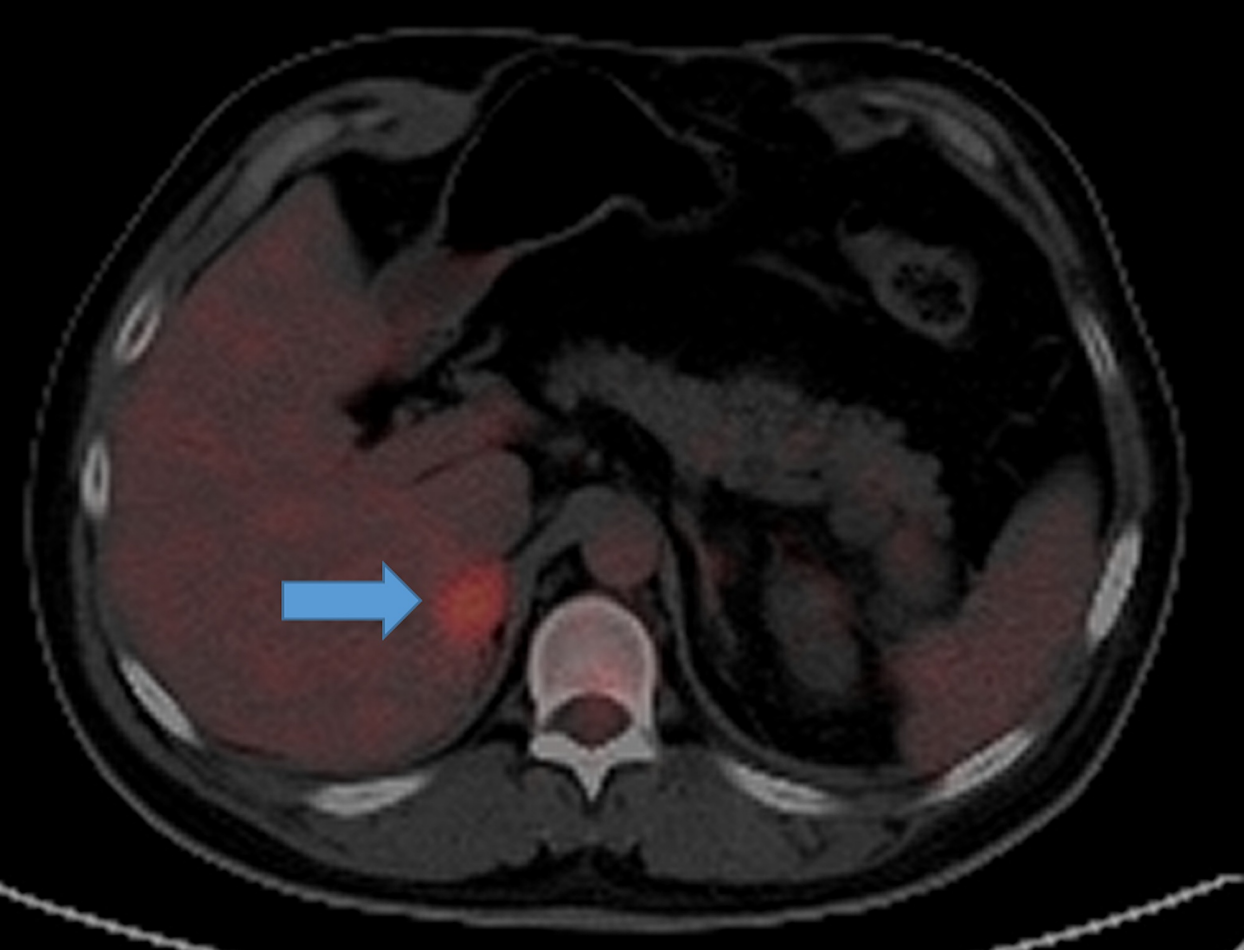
Positron emission tomography following 18F-FDG uptake An infiltrative, hyper-metabolic nodule dependent on the right adrenal gland can be seen in the image. It measured 24 x 19 mm and exhibited a soft tissue density and an SUV_max_ of 6. 8.
